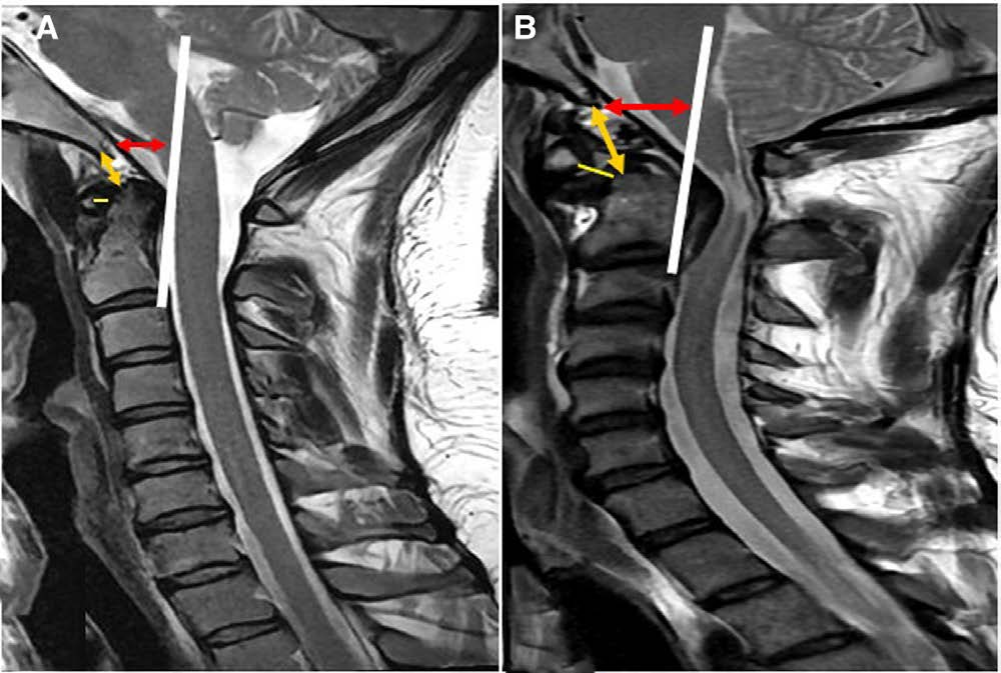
CCJ measurements: ADI (yellow line), BDI (orange line), and BAI (double-headed red arrow) in the sagittal T2-weighted MRI. (A) Sagittal T2-weighted MRI of a companion subject showing normal CCJ Indices. (B) Sagittal T2-weighted MRI of the presented patient with an old missed cervical spine injury demonstrating abnormal measurements (ADI = 6 mm, BDI = 18 mm, and BAI = 15 mm). ADI = atlanto-dental interval, BAI = basion-axial interval, BDI = basion-dense interval, CCJ = craniocervical junction, MRI = magnetic resonance imaging.
